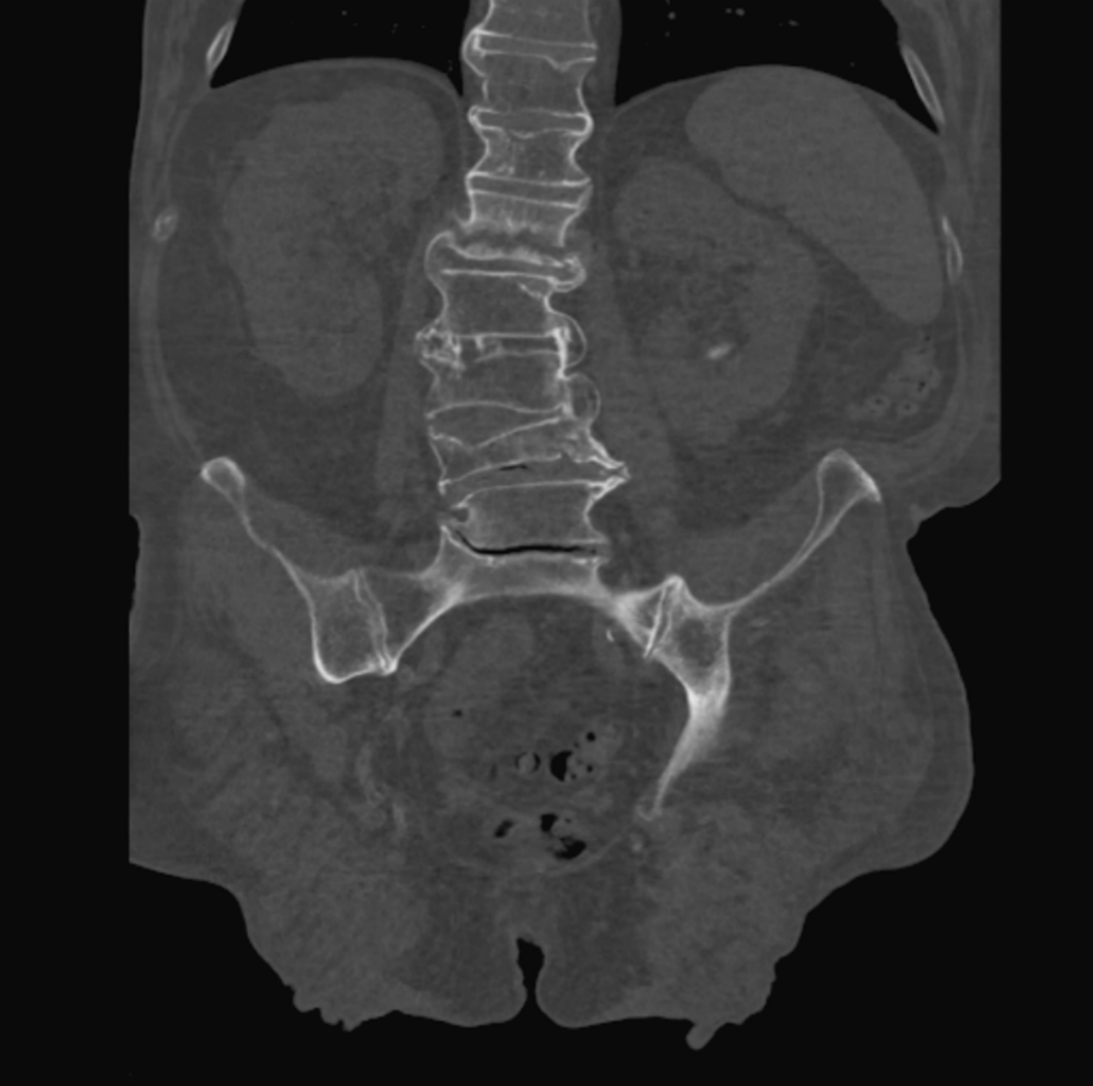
Computed tomography (CT) scan of the patient showed severe fractures of the spine.
